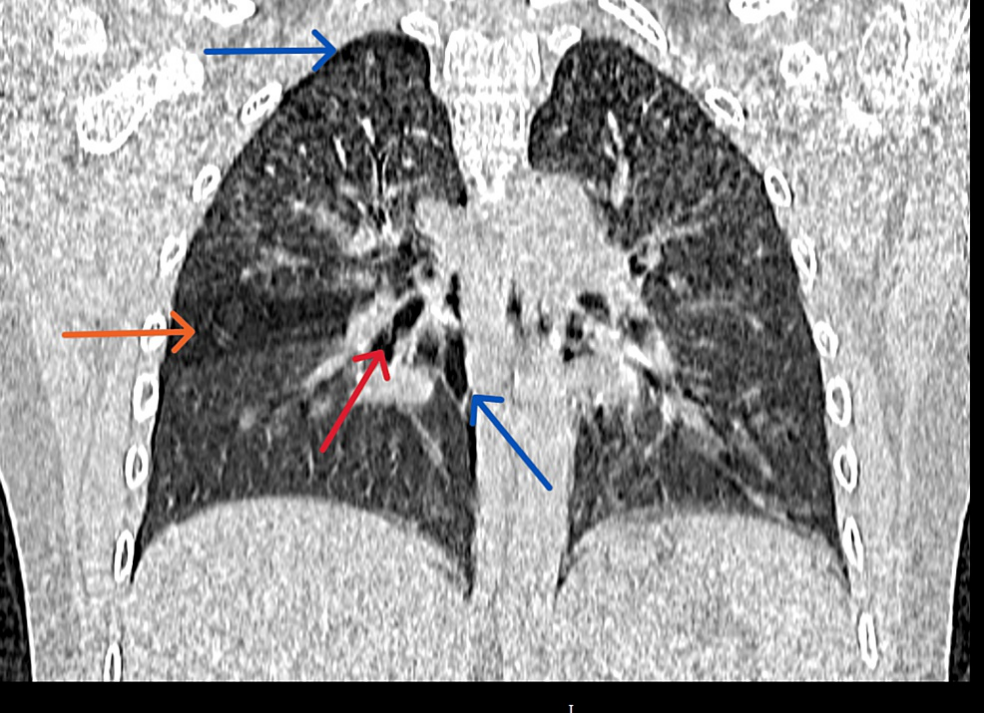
A slice of CT (coronal view) showed the foreign body (red arrow), pneumothorax, pneumomediastinum (blue arrow), and middle lobe air trapping (orange arrow).
